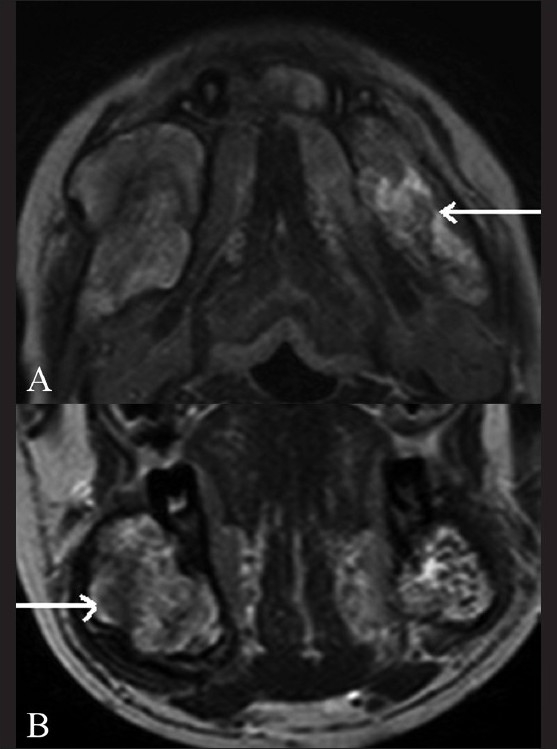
Post-embolisation T2W axial (A) and coronal (B) MRI images show thrombosis (arrow) of the AVM in the mandible and the absence of flow voids
